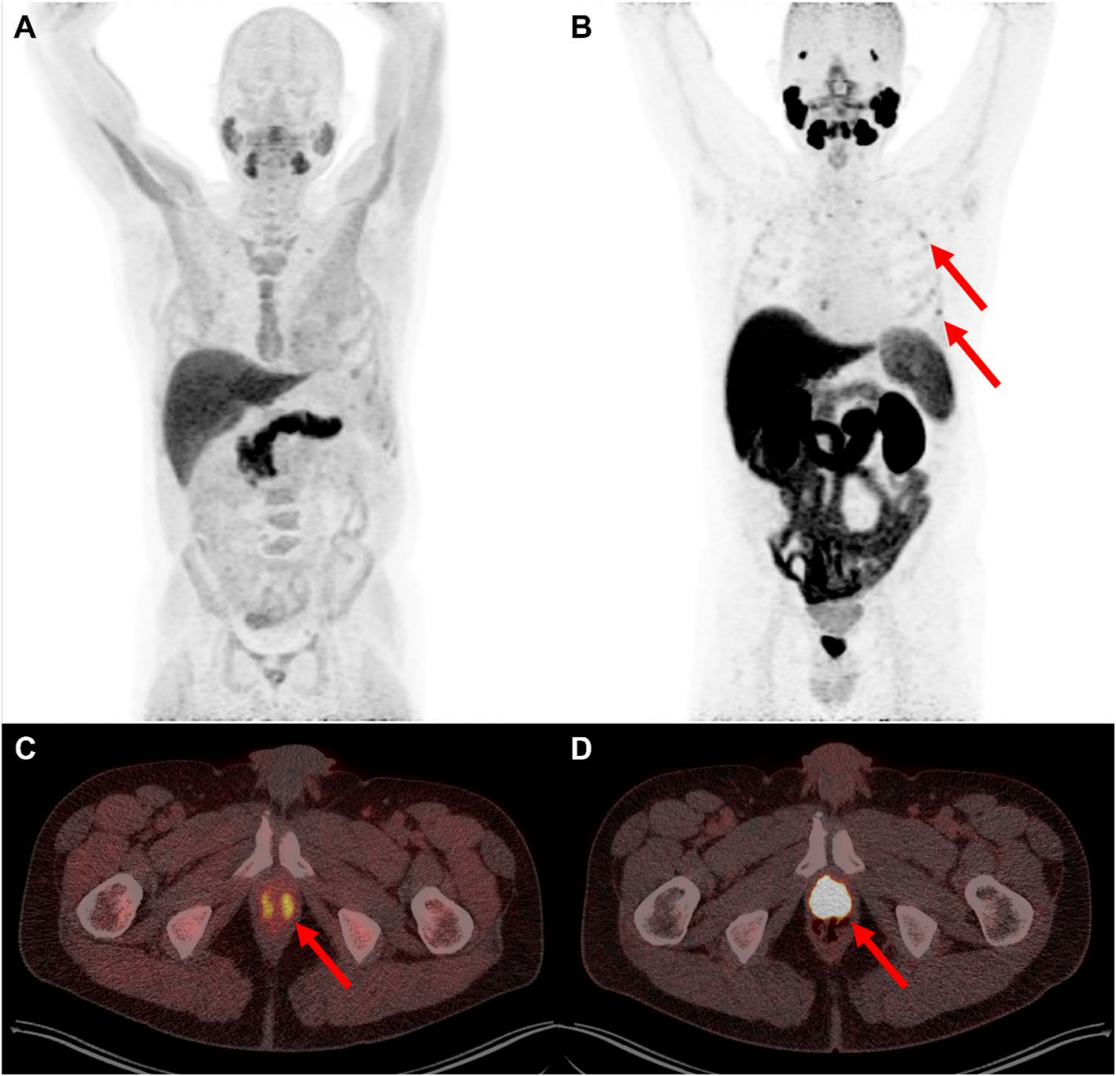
Scans with both tracers of same patient with previous therapy radical prostatectomy. [^18^F]PSMA-1007 PET/CT detecting lesion in prostate (bed) region with higher SUV_max_ than [^18^F]Fluciclovine PET/CT. [^18^F]Fluciclovine PET maximum intensity projection (MIP) image (a), no uptake in the ribs is detected. High background uptake in the bone marrow, muscles and pancreas. [^18^F]PSMA-1007 PET MIP image (b), with examples of unspecific low level bone uptake in the ribs (red arrows). Transversal slides showing lesion in the prostate (bed) region detected with [^18^F]Fluciclovine PET/CT (SUV_max_ 8.6) (c) and [^18^F]PSMA-1007 PET/CT (SUV_max_ 59.6) (d)
